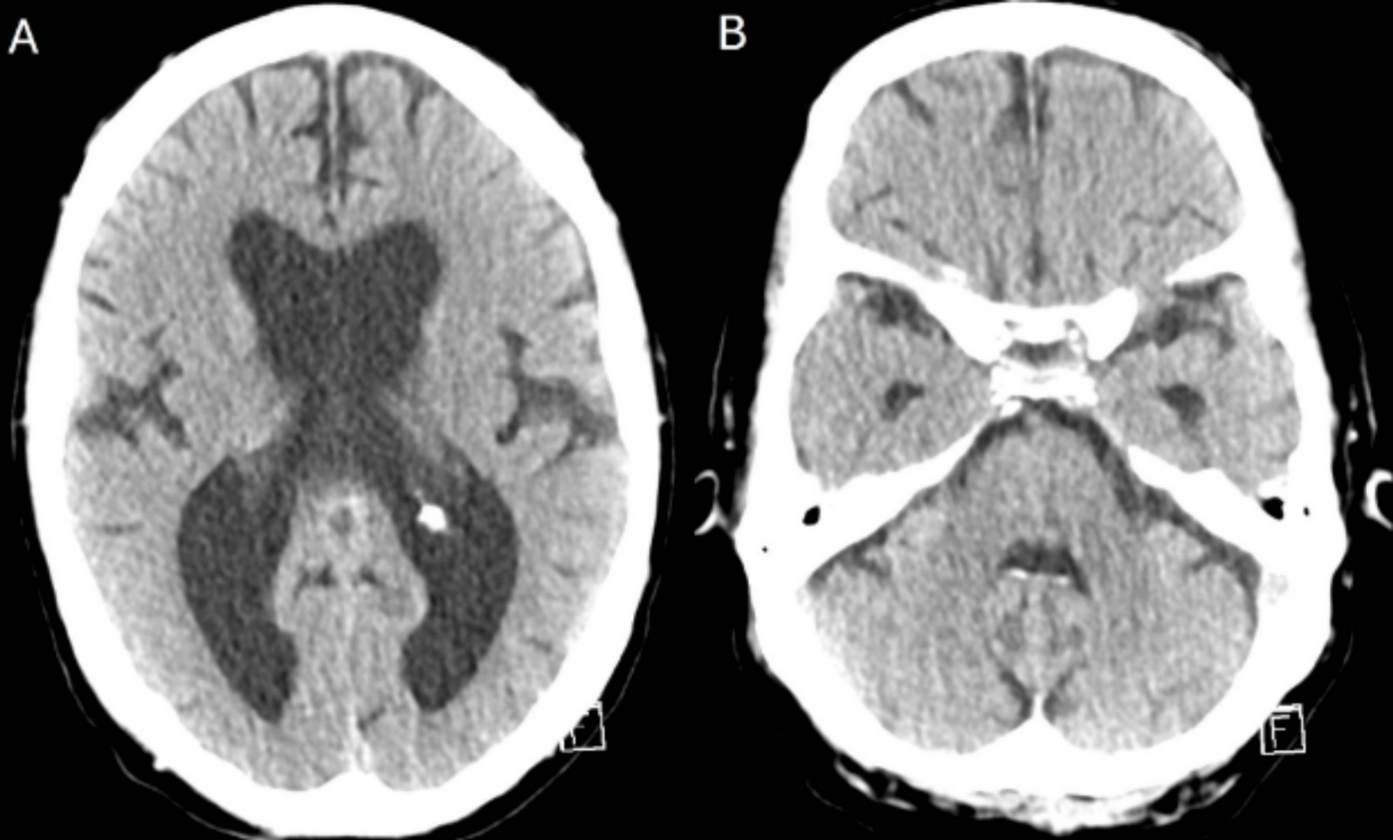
Dual-energy CT Axial images (A, B) were obtained from the dual-energy CT of the head, demonstrating the absence of hyperdensities within the ventricles and subarachnoid space.
